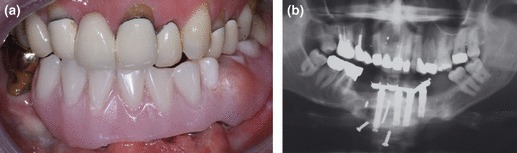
(a) This patient suffered numerous injuries subsequent to a road traffic accident approximately 25 years ago. She lost the majority of her mandibular teeth in addition to the need for grafting and fixation. Once stabilized, she was provided with an implant retained prosthesis. (b) Radiograph 20 years after initial provision. During this period, the patient presented with a variety of maintenance requirements that included clip fractures, abutment screw loosening, overdenture replacement and peri-implant mucositis.
